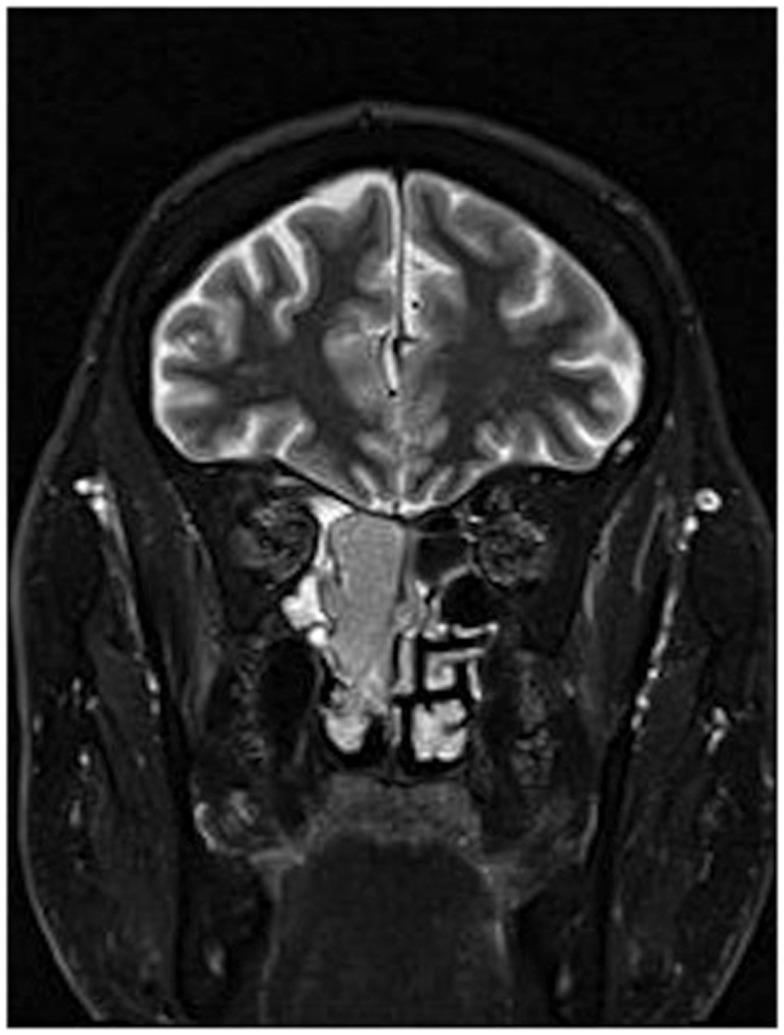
MRI of esthesioneuroblastoma/inverted papilloma collision tumor. Mass can be visualized in addition to inspissated secretions in the surrounding sinuses.
